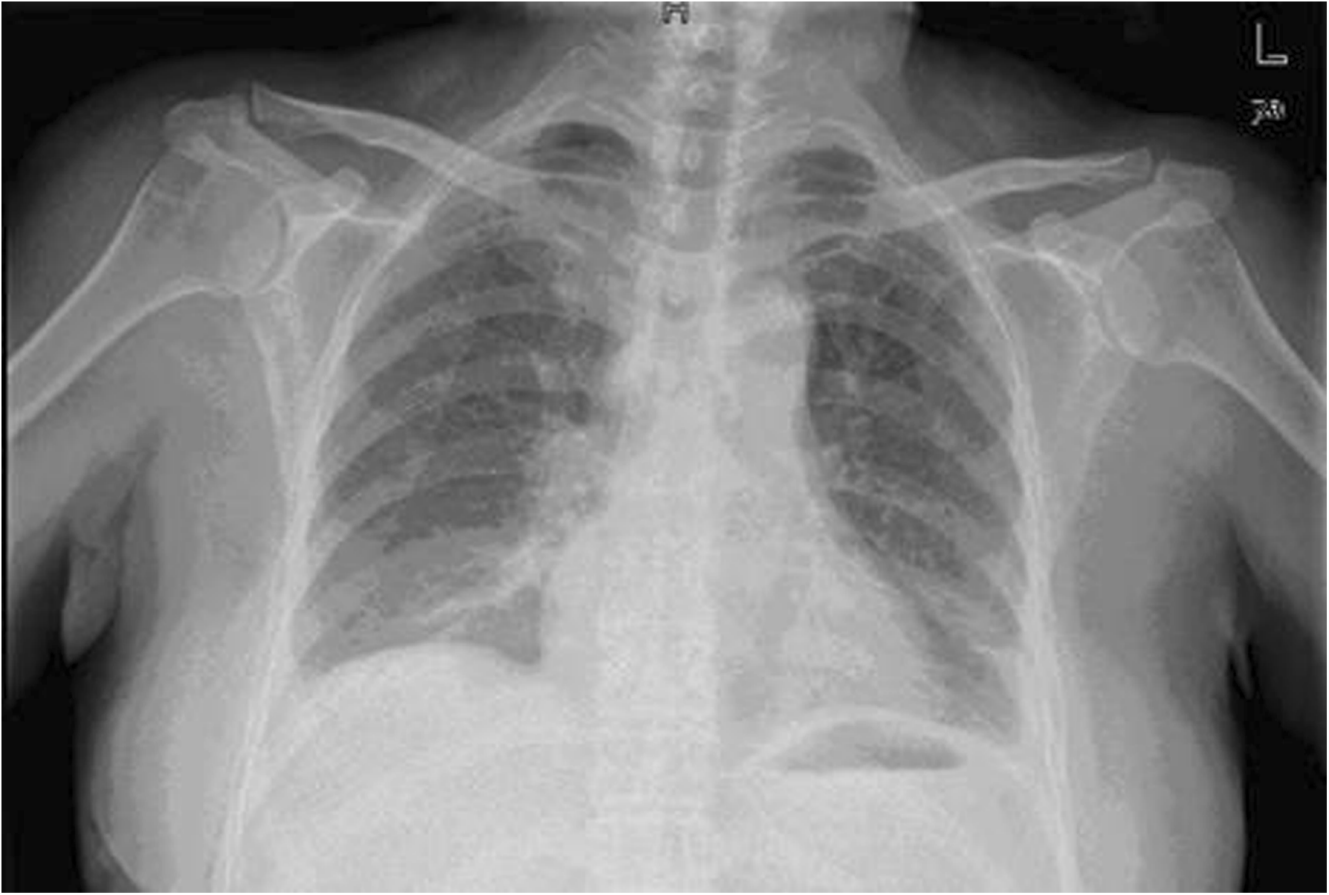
Postoperative chest radiograph
